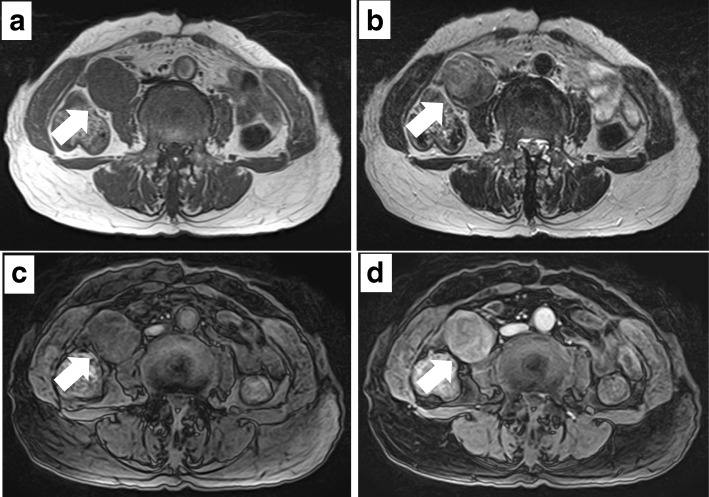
Magnetic resonance imaging of the leiomyosarcoma. a Axial T1-weighted magnetic resonance imaging (MRI) of the abdomen revealing a tumor (white arrows) with diffuse low intensity. b Axial T2-weighted MRI revealing a tumor with uniform low intensity. Early-phase (c) and late-phase (d) axial gadolinium-enhanced MRI revealing heterogeneous and incremental enhancement of the tumor
